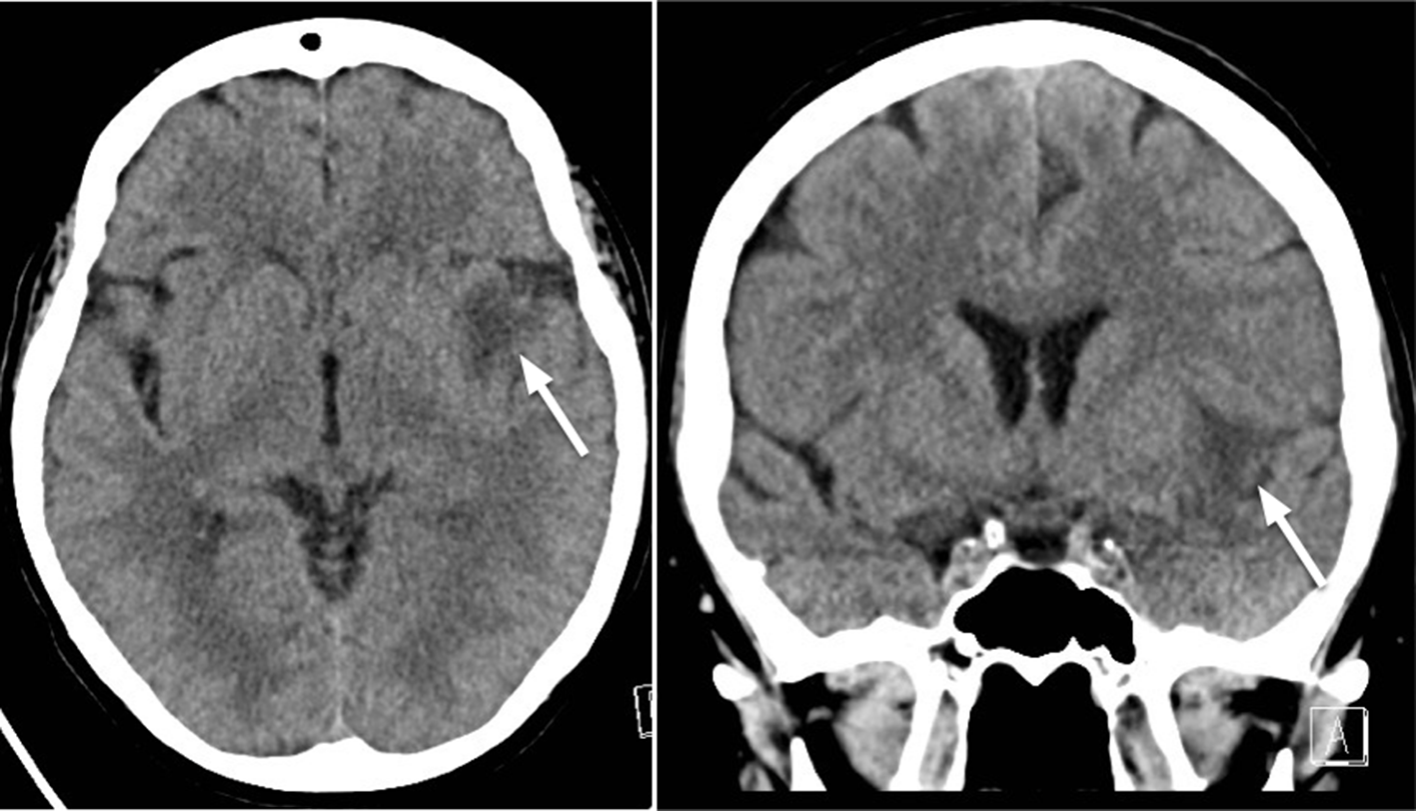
Axial (left) and coronal (right) unenhanced CT of the brain demonstrates
